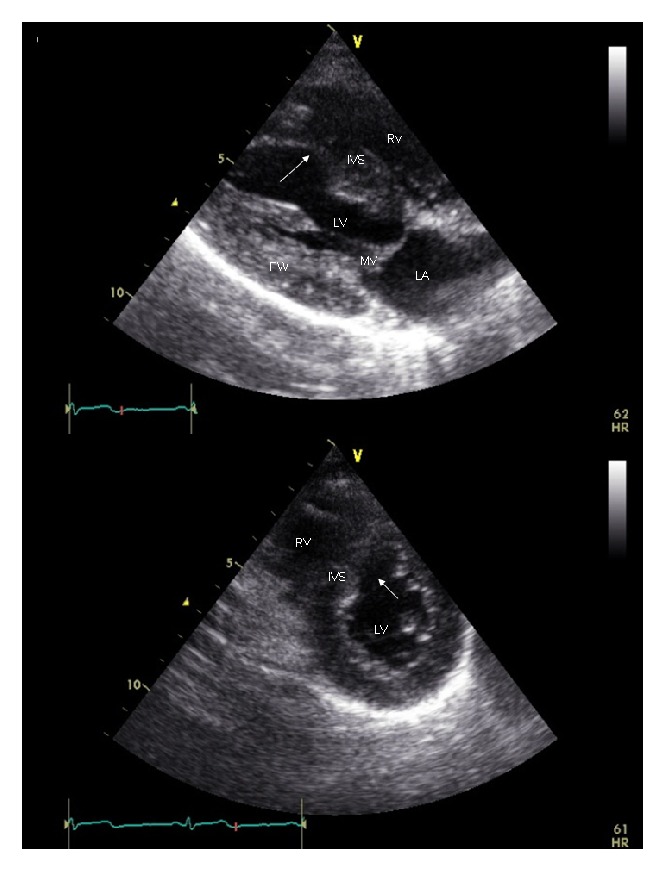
Echocardiographic images taken during ventricular systole from the parasternal long-axis view (top panel) and short-axis view (bottom panel), showing akinesia of the middle segment of the ventricular septum (arrows). Note the lack of systolic thickening of the akinetic segment, as compared to the others. IVS: interventricular septum; LA: left atrium; LV: left ventricle; MV: mitral valve; PW: posterior wall of the left ventricle; RA: right atrium; RV: right ventricle.
